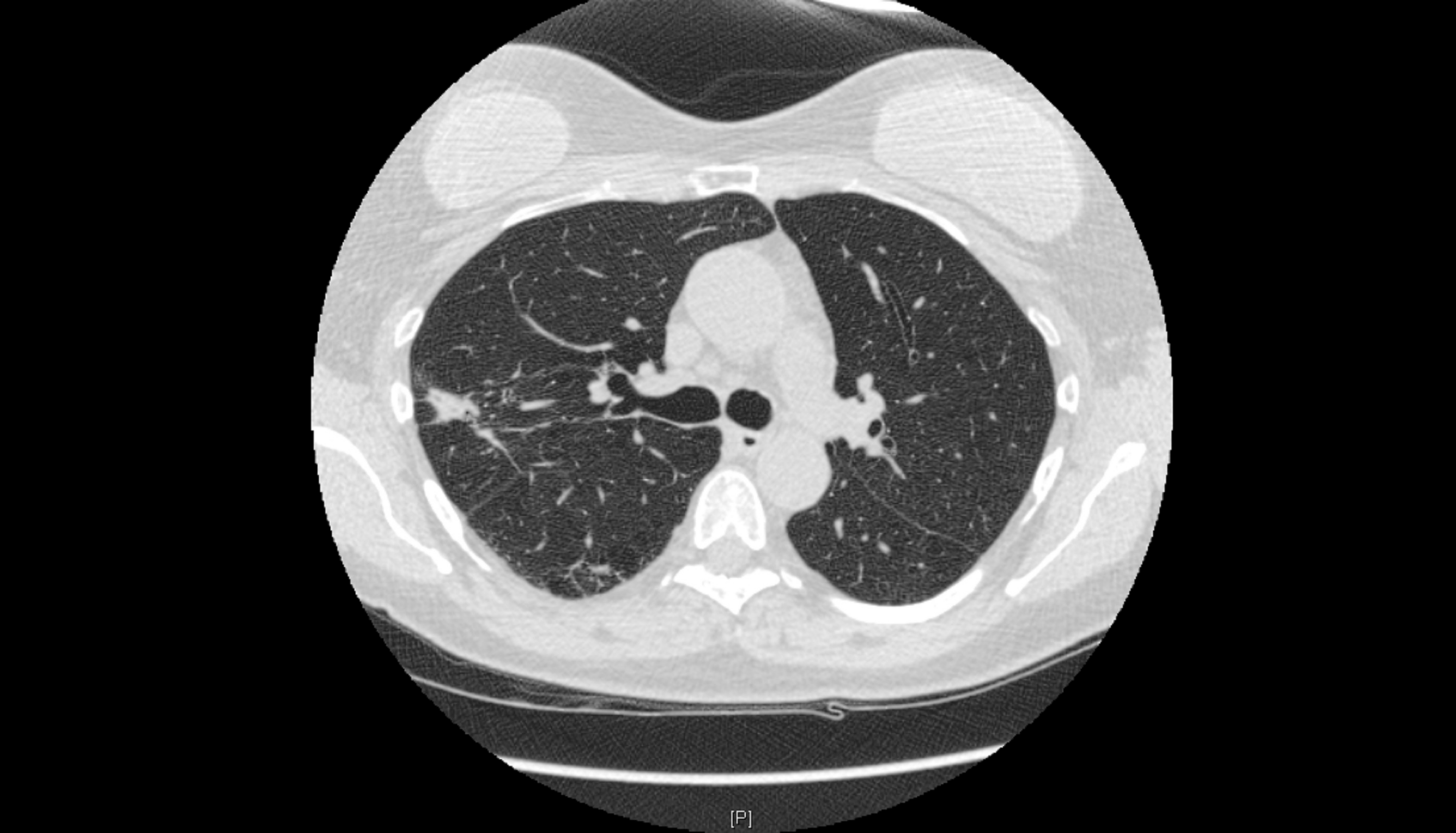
CT scan of the chest in March 2013 A solid right upper lobe 2.0 x 1.0 cm nodule is seen.
